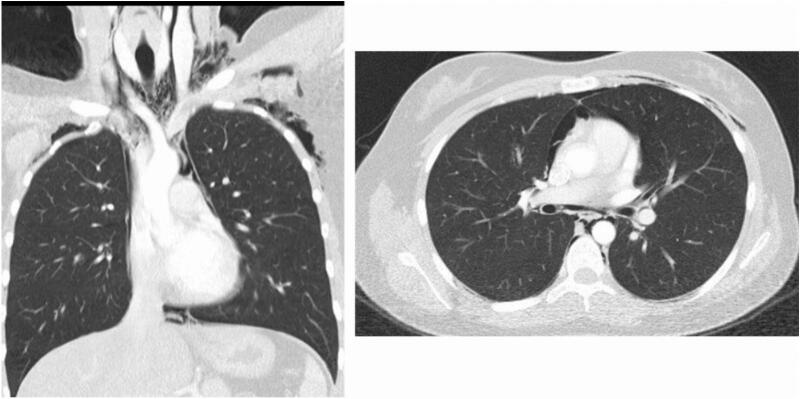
Chest CT scan. Pneumomediastinum.
